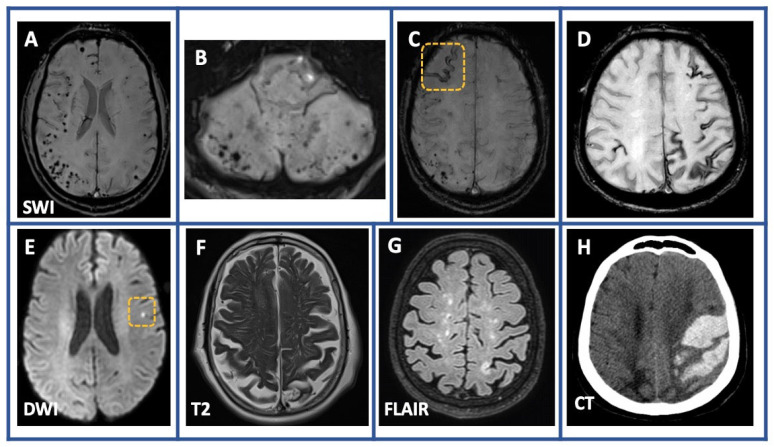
Common neuroimaging findings among patients with Cerebral amyloid angiopathy. Susceptibility-weighted imaging (SWI) sequences showing multiple cerebral (panel A) and cerebellar microbleeds (panel B) as well as focal (panel C) and disseminated (panel D) cortical superficial siderosis. Diffusion-weighted imaging (DWI) sequence (panel E) demonstrating a cortical microinfarct left parietal. Non-hemorrhagic lesions such as enlarged perivascular spaces in centrum semiovale and multispot white matter hyperintensities pattern are depicted in T2-weighted image (panel F) and 3D fluid-attenuated inversion recovery sequences (FLAIR) sequences (panel G), respectively. Large lobar intracerebral hemorrhage with characteristic finger-like projections is depicted on the baseline computed tomography of one of our patients (Panel H).
